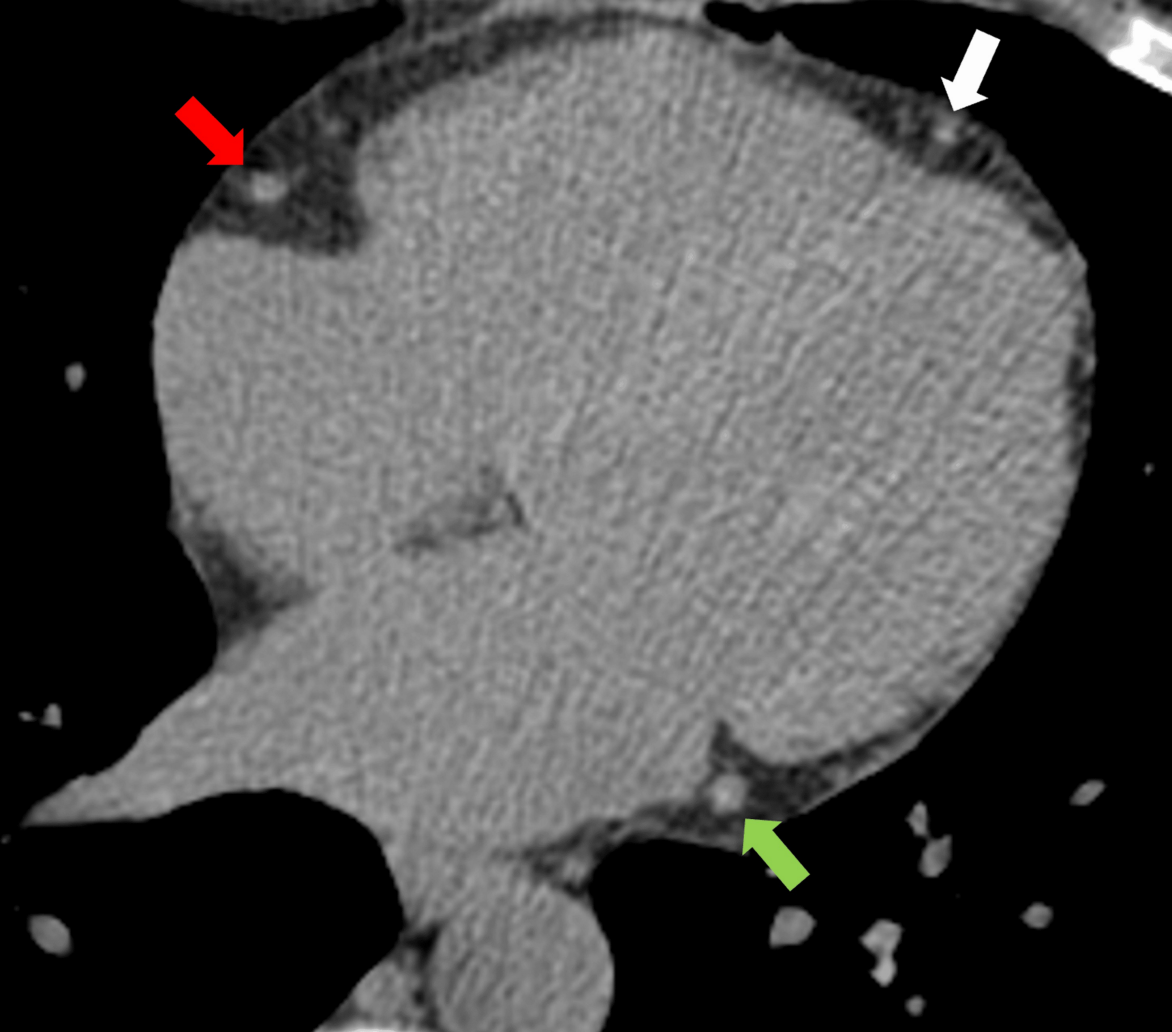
A 51-year-old female with a coronary artery calcium score of zero. Axial non-contrast cardiac-gated CT image of the heart shows no calcified atherosclerotic plaque in the left anterior descending artery (white arrow), the left circumflex artery (green arrow), or the right coronary artery (red arrow) CT: Computed tomography CT images are the property of Dr. Jordan Ditchek
